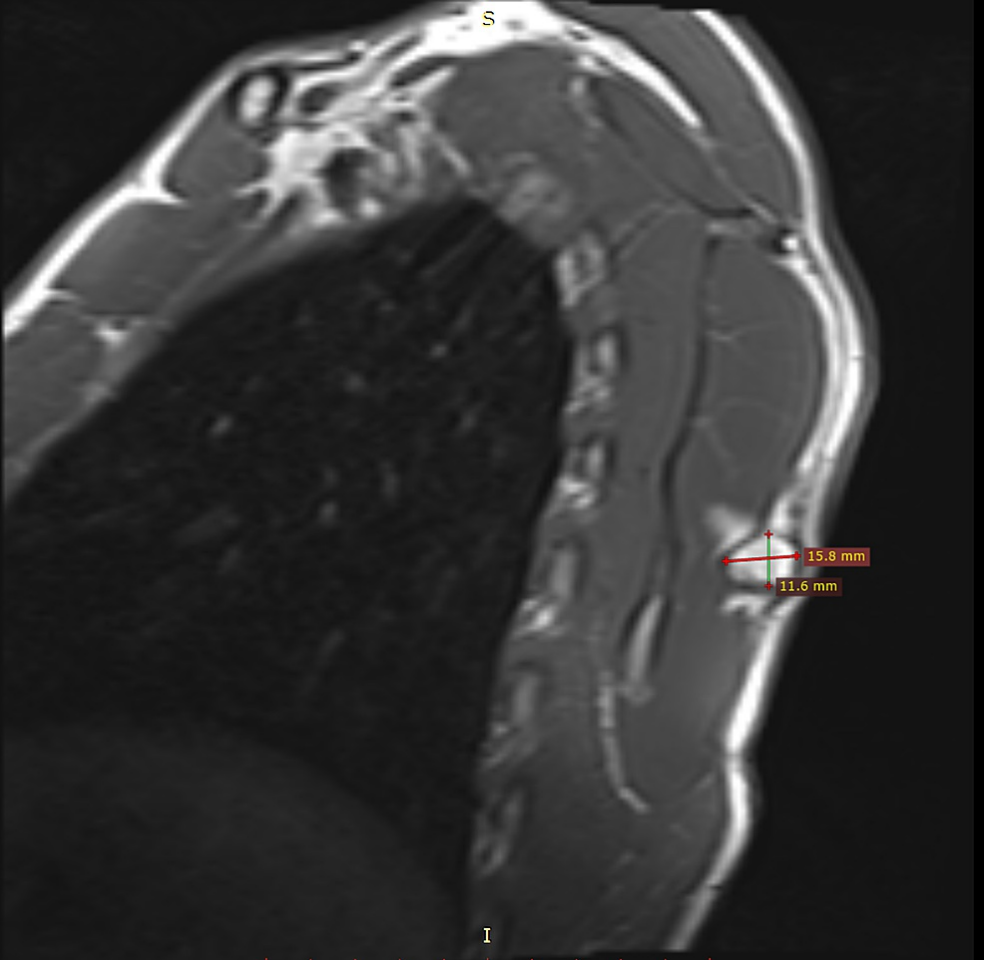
MRI sagittal view with the exostosis on the dorsal aspect of the left scapula. MRI: magnetic resonance imaging
